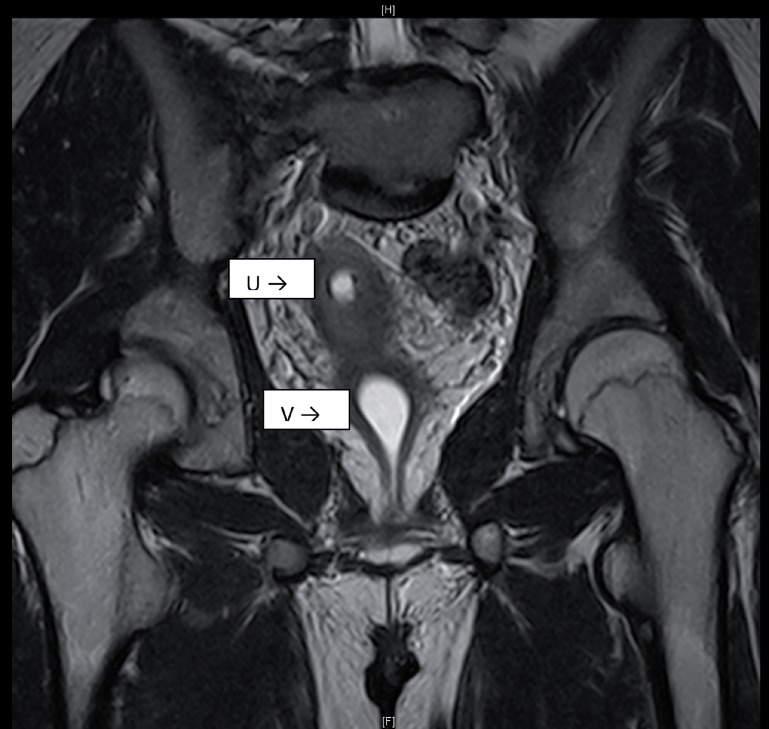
MRI of the pelvis, showing the uterus (U) and the hydrocolpos (V).
